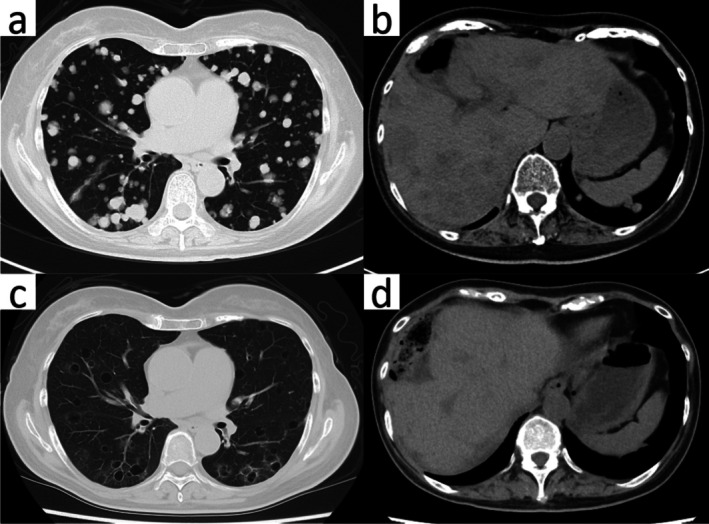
Contrast‐enhanced CT demonstrating disease at baseline and after enfortumab vedotin (EV). (a) Baseline axial lung‐window CT showing solid pulmonary metastases without cavitation. (b) Baseline abdominal CT showing hepatic metastases. (c) After two EV cycles, axial lung‐window CT showing thin‐walled cavitation of pulmonary metastases without air–fluid level. (d) Concurrent shrinkage of hepatic lesions after EV.
